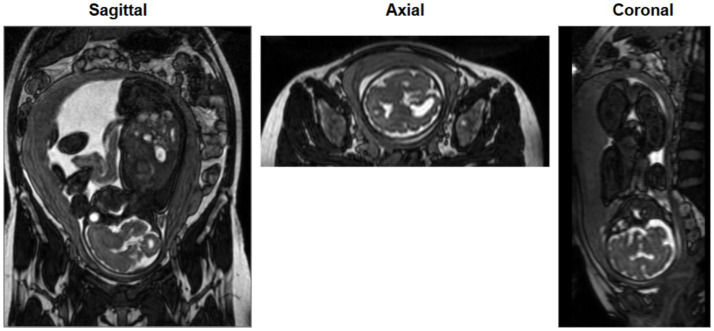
Example of SSFP whole-body fetal MRI in sagittal, axial, and coronal planes.
